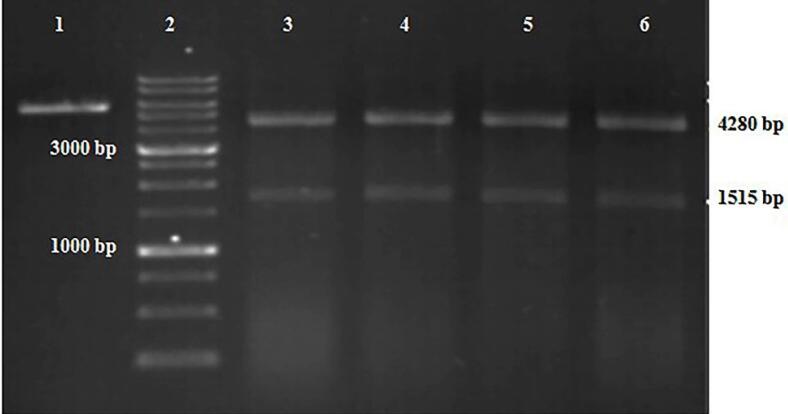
###  Optimization of PSMA-Nb expression
